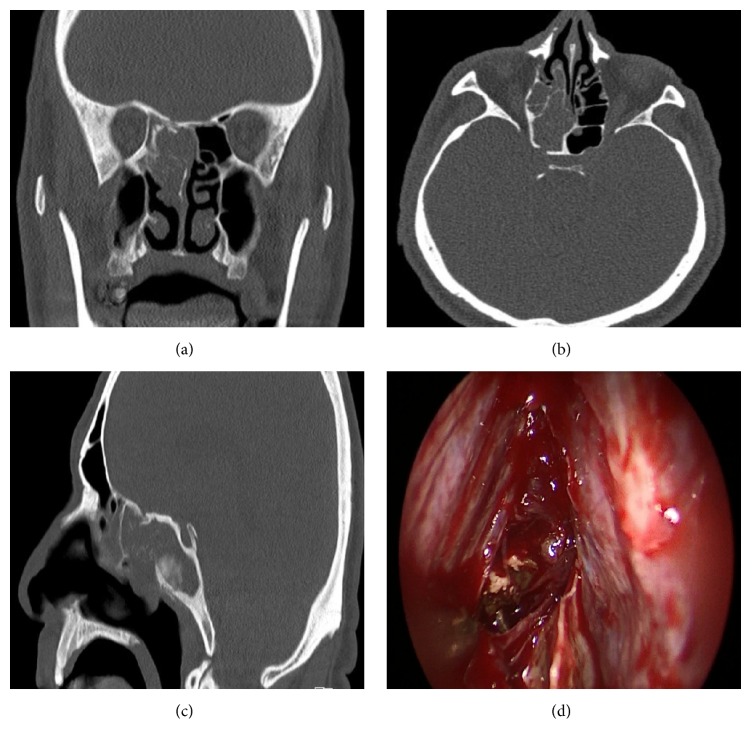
(a, b, and c) Paranasal sinus computed tomography of a hypodense mass filling the right sphenoid sinus (a-coronal, b-axial c-sagittal planes). (d) Endoscopic view of the caseous material filling the right sphenoid sinus.
